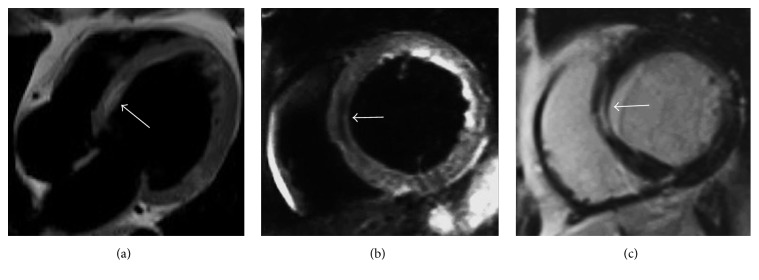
CMR examination in a 62-year-old woman with idiopathic dilatative cardiomyopathy. Four-chamber T1-weighted black blood image (a) demonstrates left ventricular chamber enlargement with an intramyocardial hyperintense stripe in the interventricular septum. Short axis black blood T2-weighted with fat suppression (b) shows a signal drop of the stripe, confirming the presence of intramyocardial fat. On short axis late gadolinium enhancement T1-weighted sequence (c) the adipose tissue location corresponds to myocardial enhancement due to concomitant intramyocardial fibrosis with mesocardial distribution within the interventricular septum.
